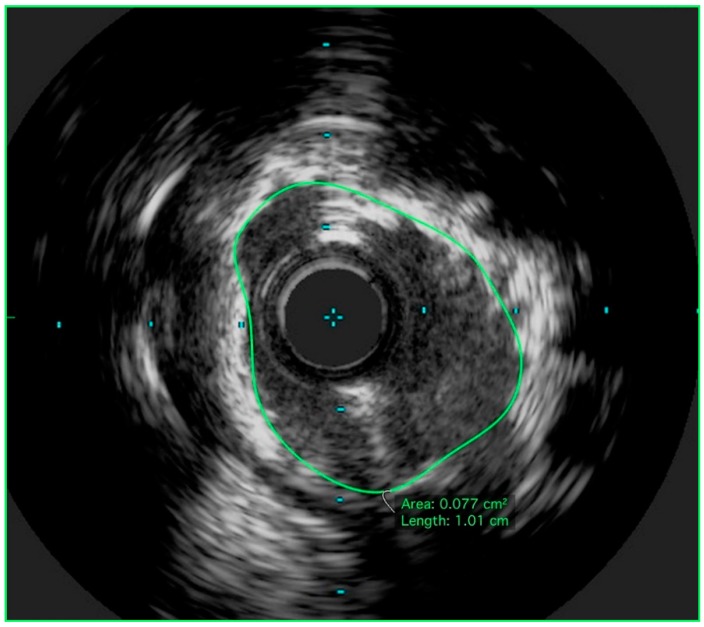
Post-orbital atherectomy IVUS of LMCA. IVUS of the LMCA with a 40 MHz imaging catheter performed after orbital atherectomy revealing 270 degrees of calcium and a minimal luminal area of 7.7 mm^2^. IVUS: Intravascular ultrasound; LMCA: Left main coronary artery.
